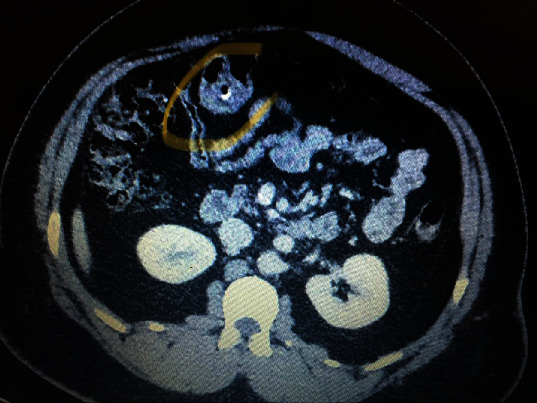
CT scan of case number 1.
